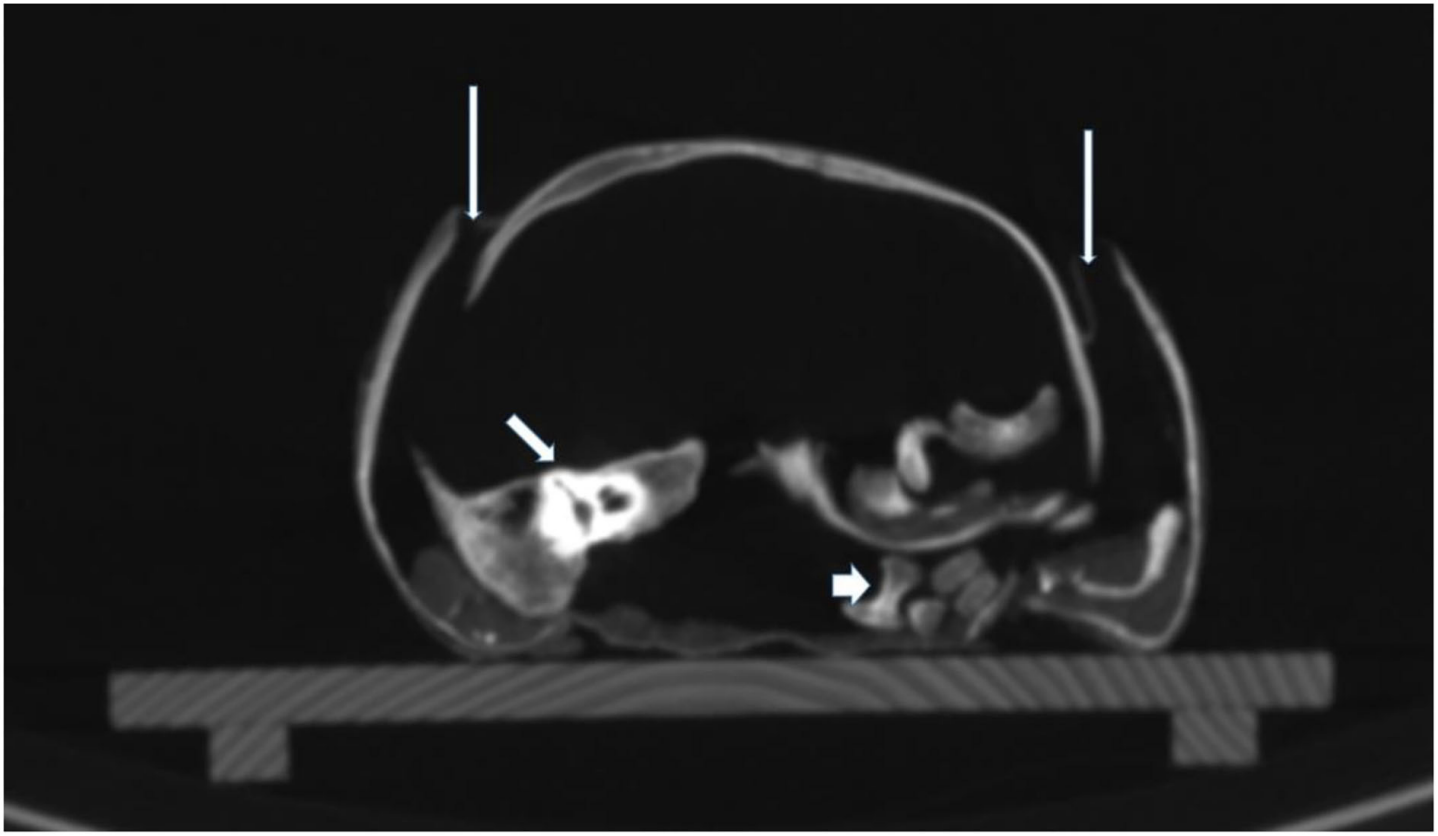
CT scan of the skull base. On cross-sections, the extensive dislocation of the bones of the skull base is visible. Thin arrows: disruption of sutures; middle arrow: dislocated right petrous bone; thick arrow: dislocated parts of vertebral bodies.
